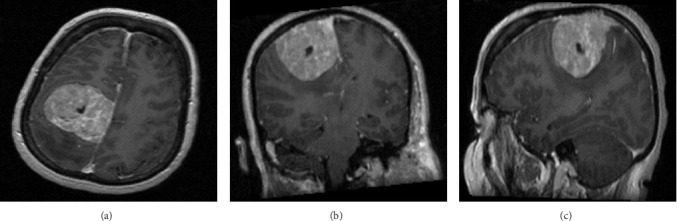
(A–C) Axial, coronal, and sagittal brain MRI post gadolinium administration demonstrating a right parasagittal extra-axial hyper-intense lesion measuring 5 cm × 5 cm × 4.8 cm in AP, transverse, and craniocaudally dimensions, respectively. The lesion is well-defined, oval-shaped, and shows avid enhancement after gadolinium administration. The lesion is causing focal mass effect in the form of compression of the ipsilateral ventricle, midline shift of about 5 mm, and subfalcine herniation. MRI, magnetic resonance imaging.
